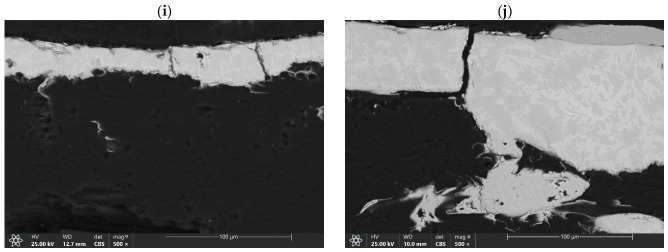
Cross-sectional SEM images of Si–Me infiltration coatings on C/C (Me = Ta, Mo, Ti, W, Zr) in the as-infiltrated state (left column) and after ultrasonic atomization (right column): (a,b) Si:Ta 1:1, (c,d) Si:Mo 1:4, (e,f) Si:Ti 1:1, (g,h) Si:W 1:5, (i,j) Si:Zr 1:5.
